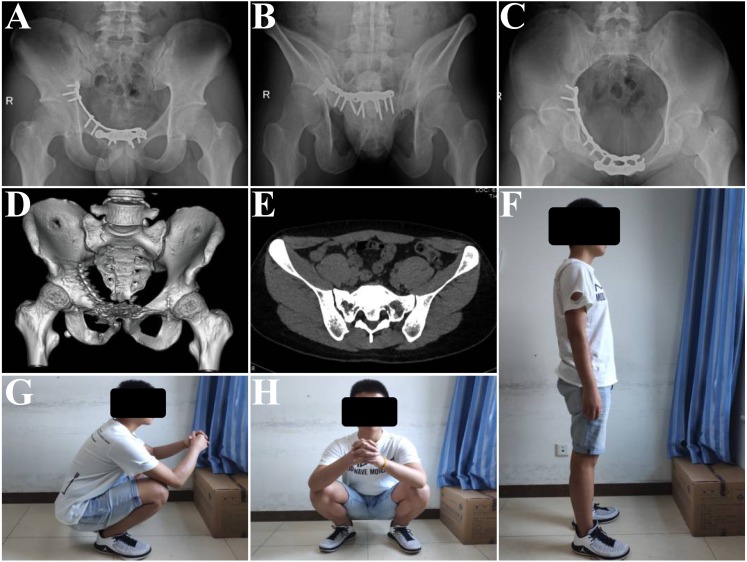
The fracture is united by the follow-up, and the Majeed score is 91 (excellent outcome). (A) The anterior-posterior plain at 5 months postoperatively. (B) The outlet plain at 5 months postoperatively. (C) The inlet plain at 5 months postoperatively. (D) Complete healing of the fracture. (E) Bone union on the axial CT scan; there is no late displacement. (F–H) The patients’ function ability in different positions.
